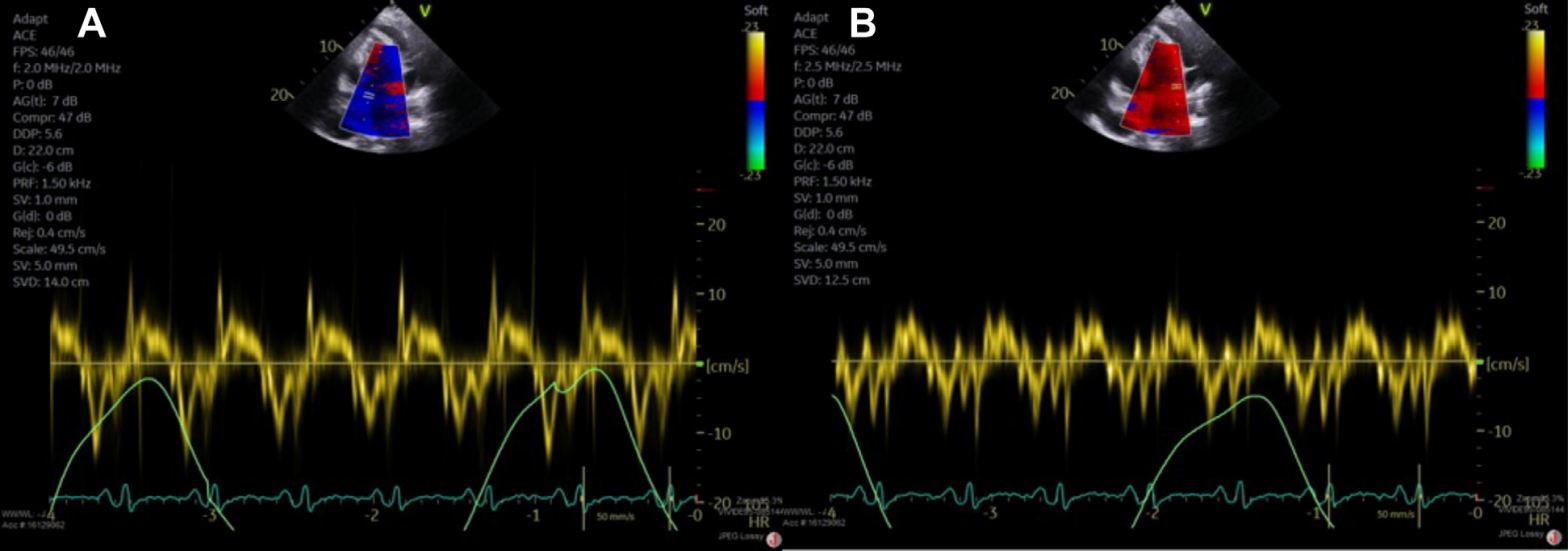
Transthoracic Echocardiogram With Tissue Doppler Echocardiogram demonstrating elevated medial mitral annular eʹ velocities greater than the lateral annular eʹ velocities, consistent with annulus reversus, and early tethering of the lateral mitral annulus with acute constrictive pericarditis.
